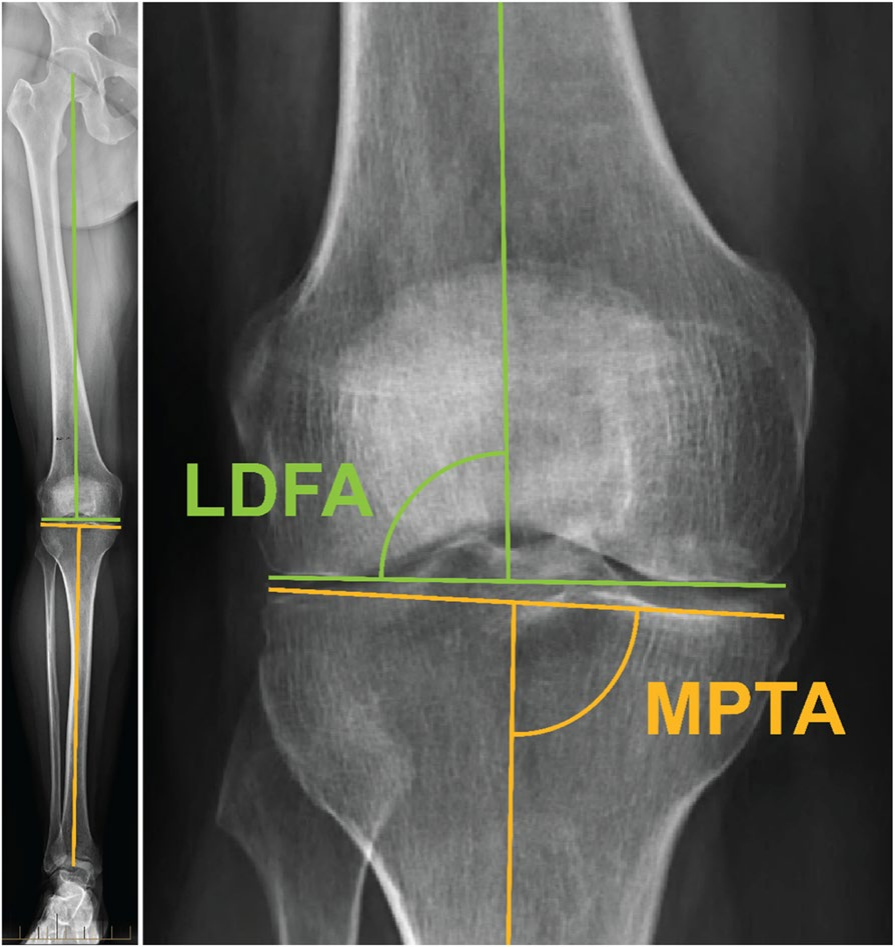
MPTA and LDFA measurement on long leg radiograph (LLR)
